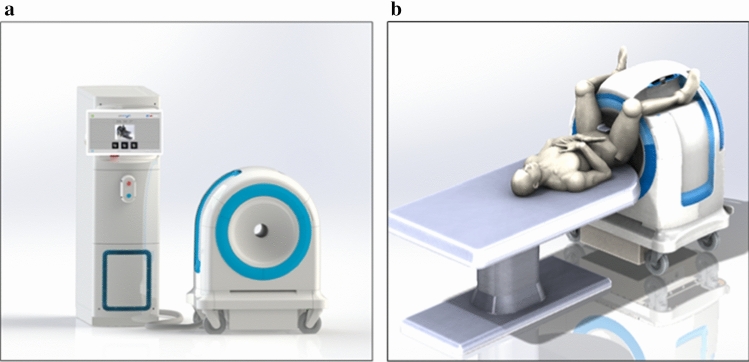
a The low-field MRI system. The auxiliary cart (left) houses the electrical and electronic components, such as the computer, programmable logic controller, power distribution unit and amplifiers. The magnet cart (right) houses the magnet, gradient coils and transmission coil and attaches to the receive coil. b The scanning position is a lithotomy position with the patient’s legs around the magnet cart
